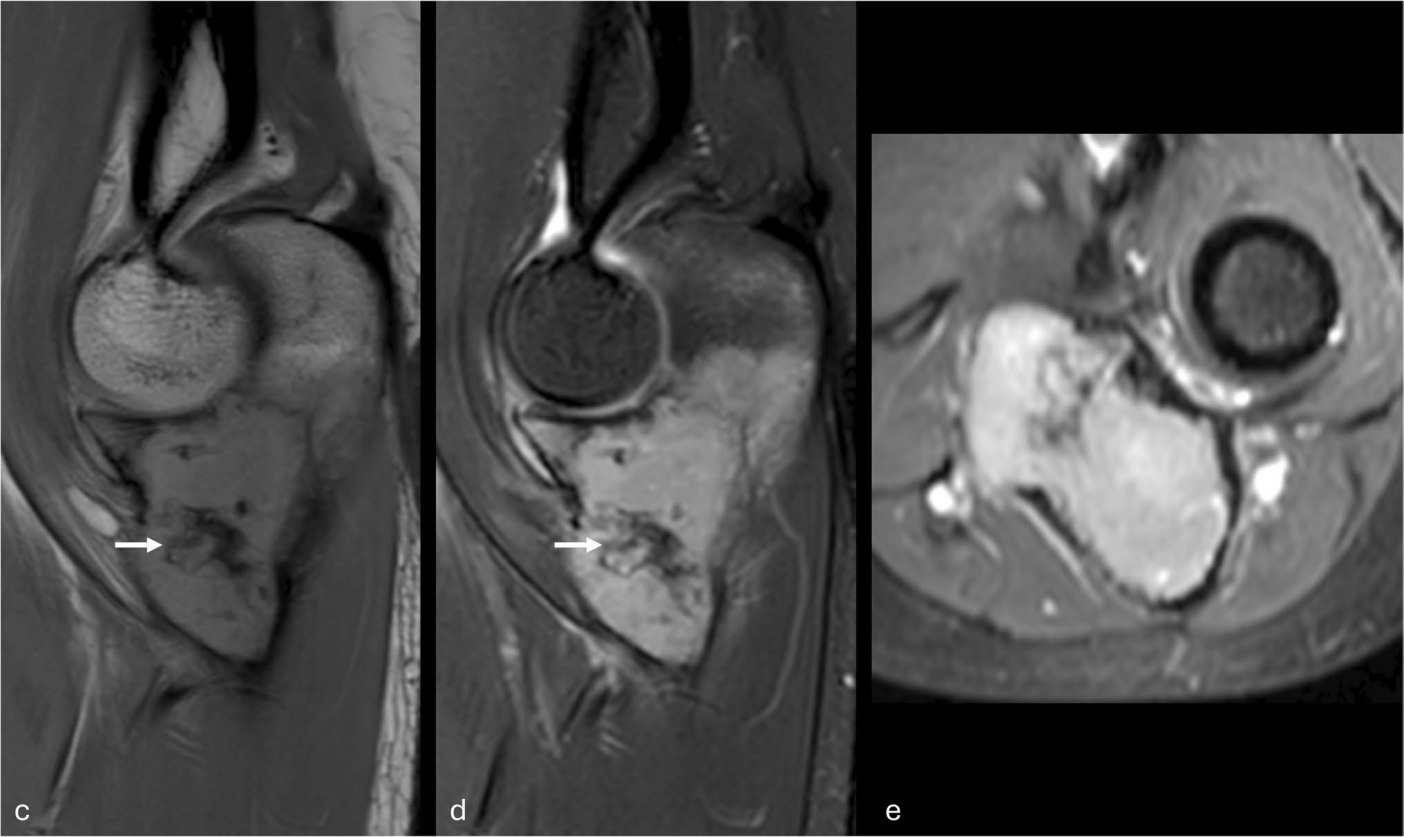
MR images of the left proximal ulna. Coronal T1 (a) and proton density (PD) fat saturated (FS) images (b) show a well-defined, expansile lesion which is mildly hyperintense on T1-weighted and markedly hyperintense on PDFS scans. Sagittal PD (c) and T2FS images (d) show a moderately hyperintense lesion extending to the subarticular bone, with a prominent central area (arrows) and small punctate foci of low signal intensity consistent with calcification. Axial T1-weighted FS post-contrast (e) image shows a diffusely enhancing lesion with eccentric low signal anteriorly due to calcification. The anterior cortex is markedly thinned, but no soft tissue extension is seen
